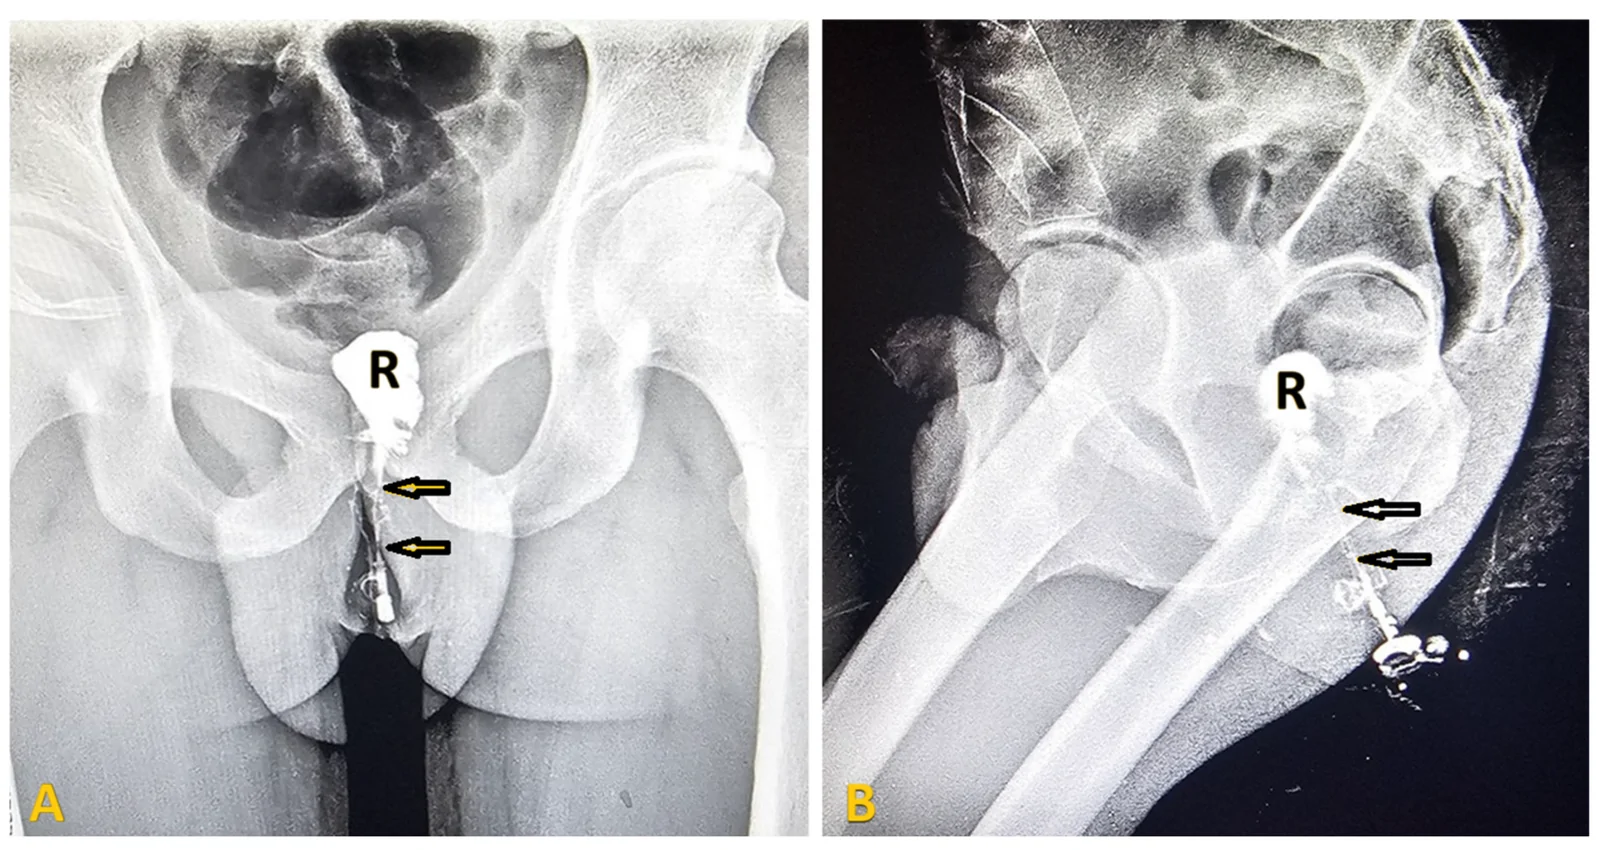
Selected X-ray fistulogram showing a high perianal fistula showing the contrast-filled fistulous tract (arrows) and contrast leakage into the rectum (R) on (A) anteroposterior and (B) lateral views.
